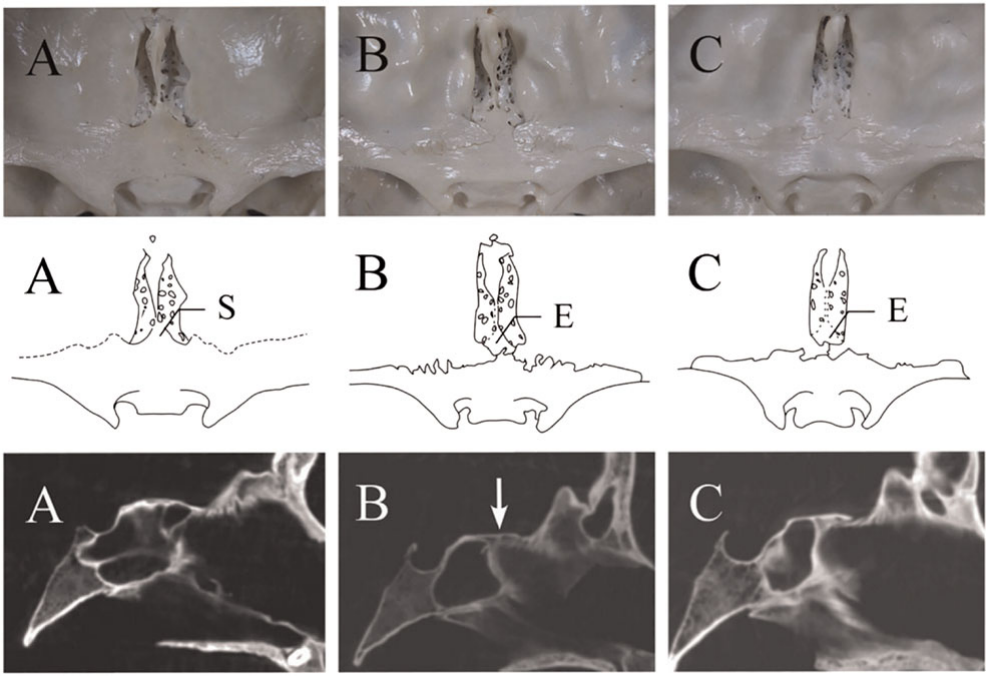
Morphological variations of the anterior cranial fossa in adult skulls. Upper row shows endocranial photographs, middle row shows traced images, and lower row shows dental cone‐beam computed tomography (CT) images in the median sagittal plane. (A) Triangular protrusion of the sphenoidal body projecting towards the ethmoid bone. Distances from the posterior border of the ethmoid bone to the lesser wing of sphenoid bone were varied. The spheno‐ethmoidal suture is not observed on CT images. (B) The anterior border of the median part of the protrusion of the sphenoid body is straight. The spheno‐ethmoidal suture is clearly evident on CT images (arrow). (C) Frontal bone intervenes between the ethmoid and sphenoid bones. The boundary of the three bones is unclear on CT images
